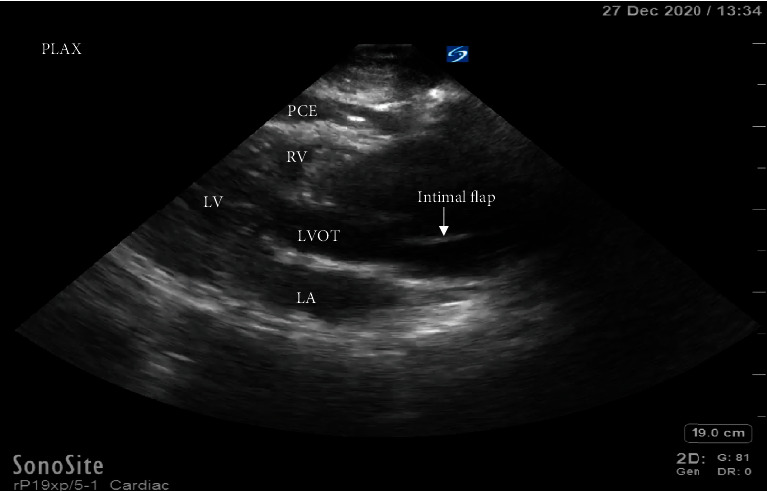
Parasternal long axis displaying the intimal flap within the aortic outflow tract. LA: left atrium; LV: left ventricle; RV: right ventricle; PCE: pericardial effusion; LVOT: left ventricular outflow tract.
